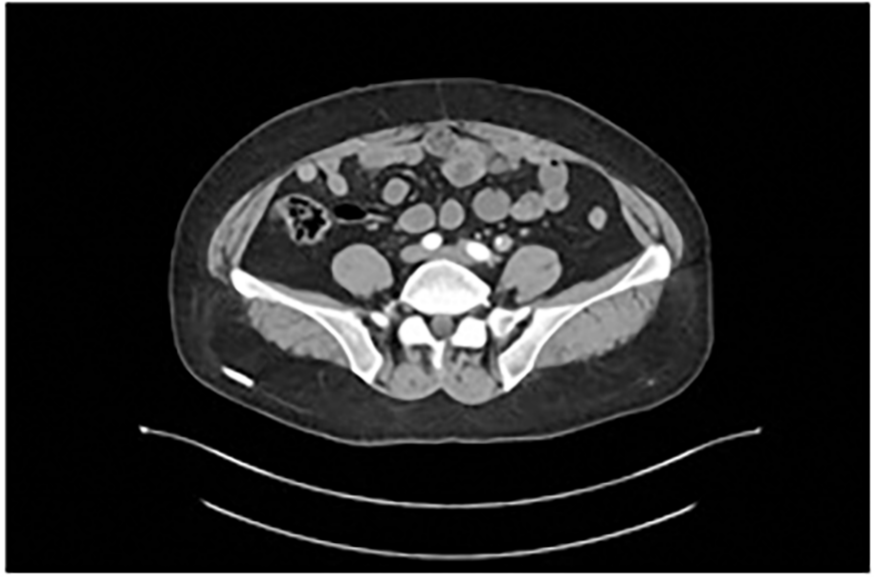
Contrast-enhanced abdominopelvic CT demonstrates circumferential thickening of the distal left ureter (1.8×1.5 cm), associated with proximal hydroureter.
